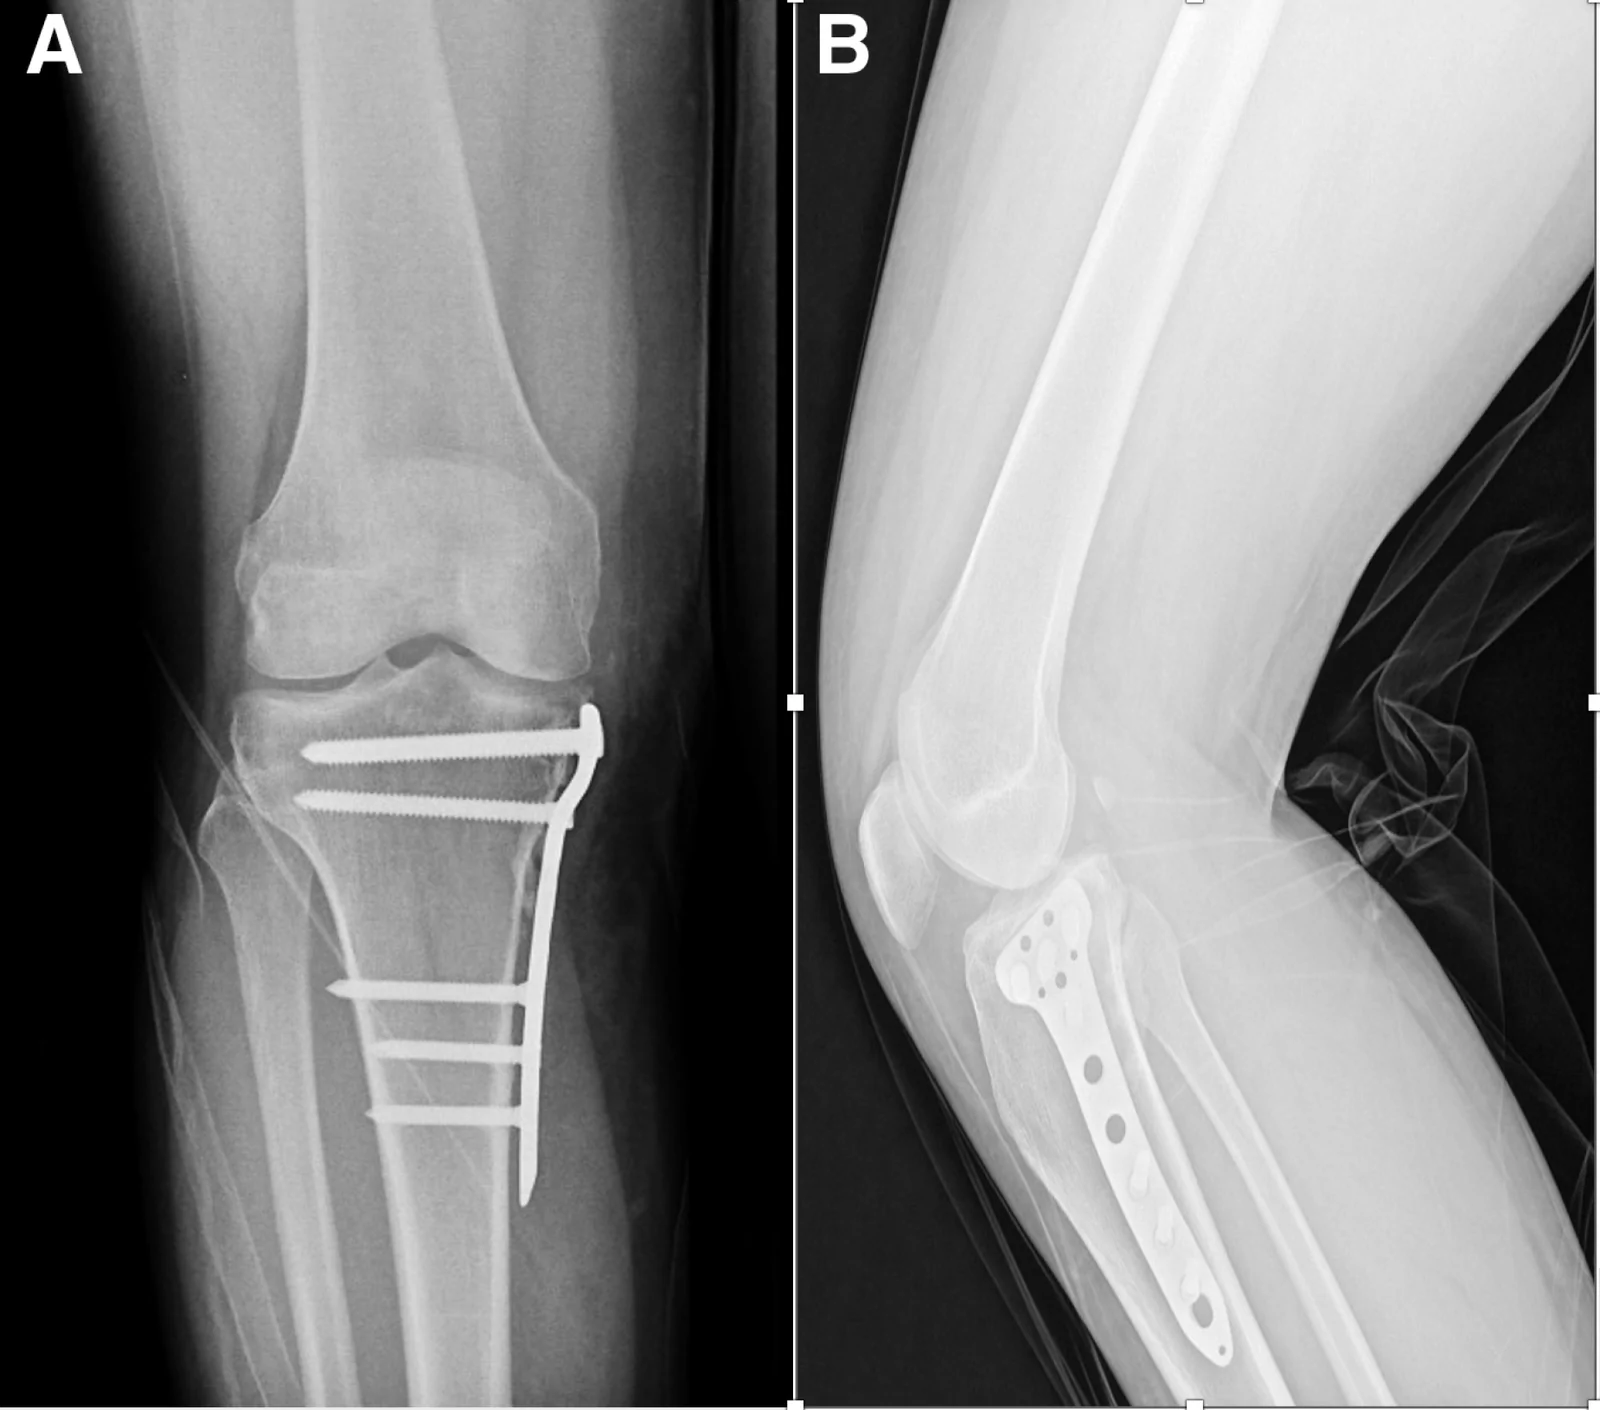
Twelve-month postoperative radiographs. (A) AP radiograph demonstrating complete fracture union, maintained anatomic reduction, and stable implant position; (B) lateral radiograph demonstrating preservation of articular congruity without secondary collapse, loss of reduction, or implant-related complications. AP = anteroposterior.
